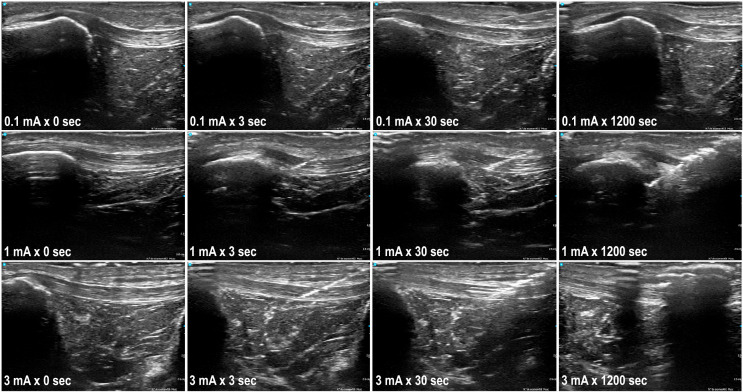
Gas effect of percutaneous electrolysis in ultrasound with different combinations of time and intensity.
